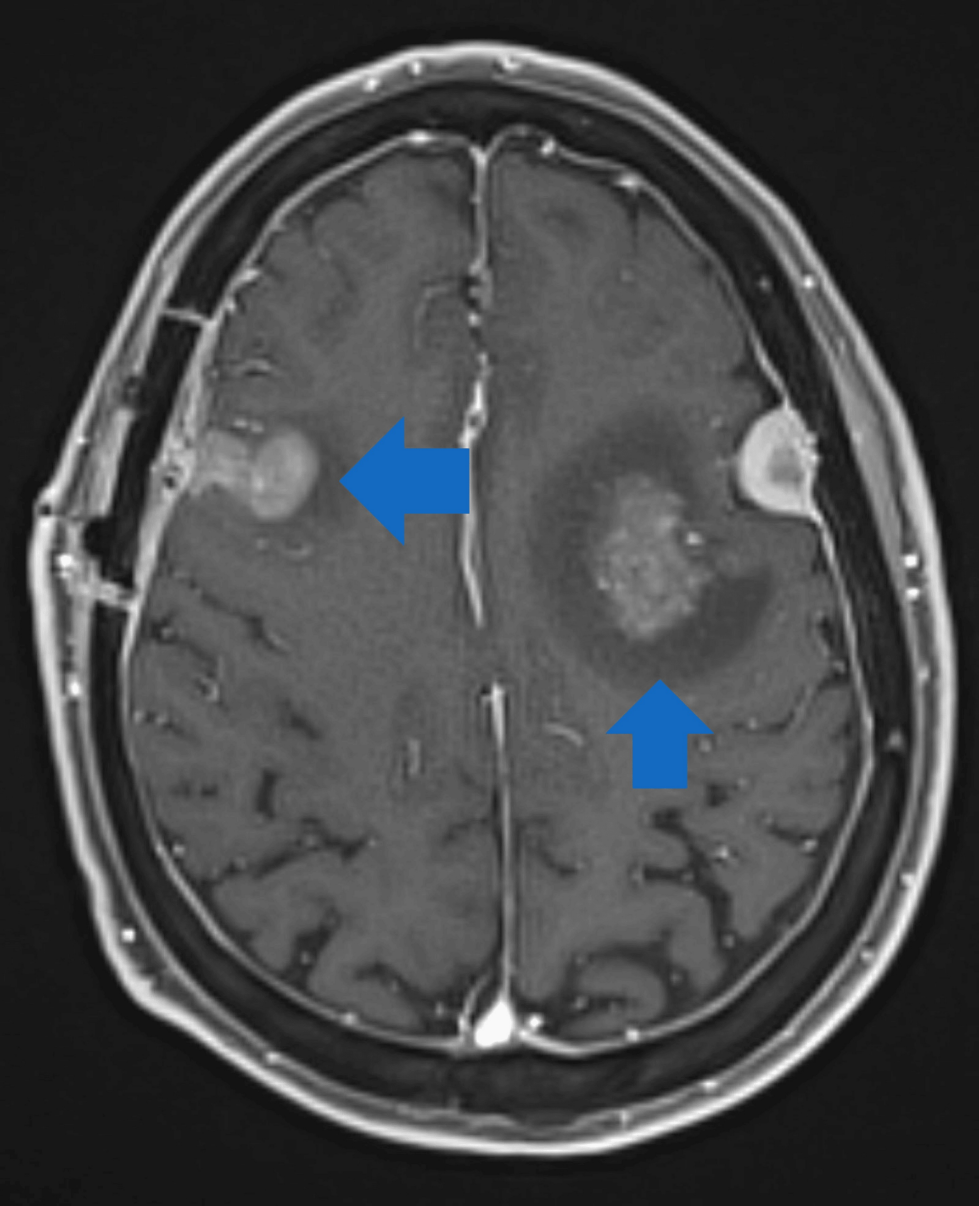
T1-weighted post-contrast MRI of the brain in patient 2 with SCNSL It shows a left posterior frontal lobe lesion involving the precentral gyrus, as well as a right frontal lobe lesion with a surrounding hemosiderin ring and associated vasogenic edema. SCNSL = secondary central nervous system lymphoma
